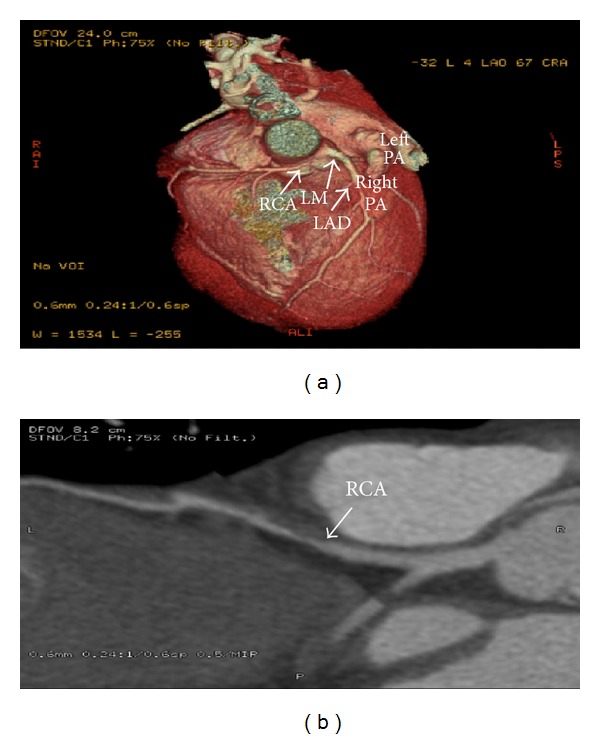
(a) Cardiac CT angiography with 3D reconstruction with digital subtraction of pulmonary arteries. (b) Cardiac CT angiography.
